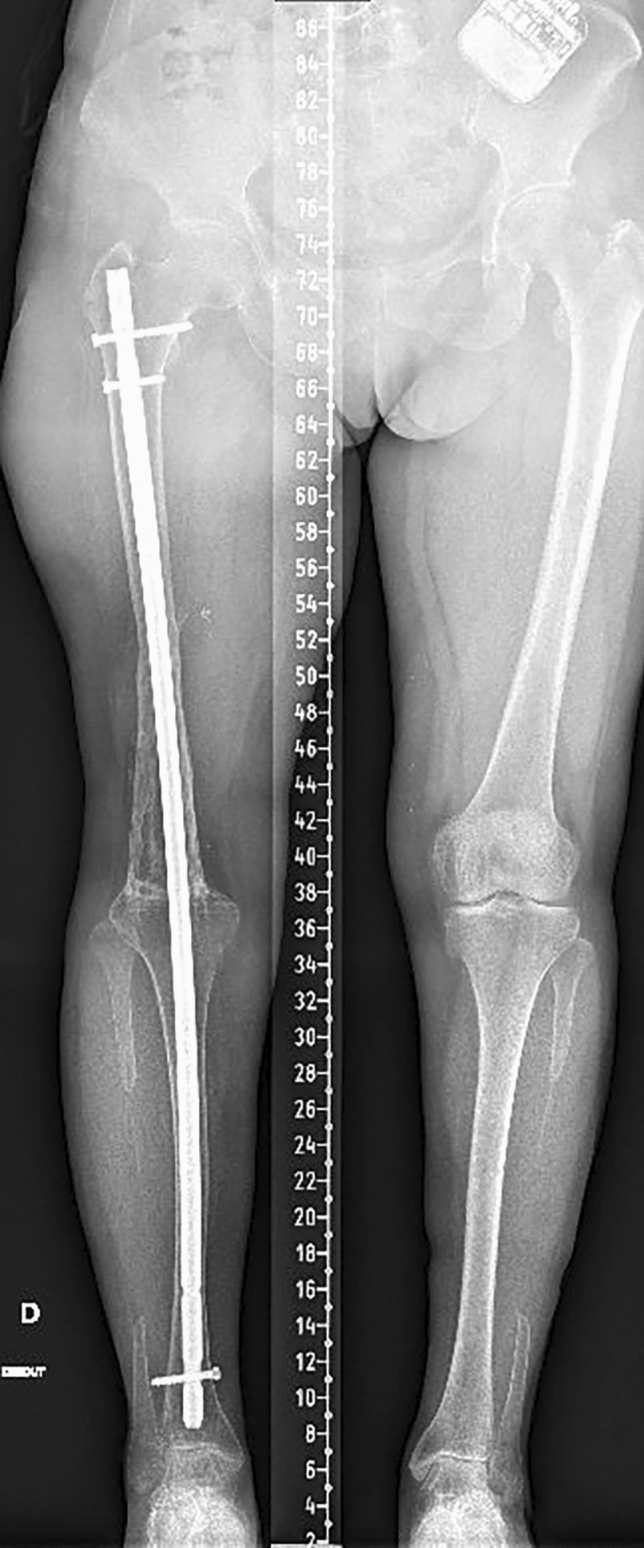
Standing whole leg radiograph of KA using T2AN at 1 year
